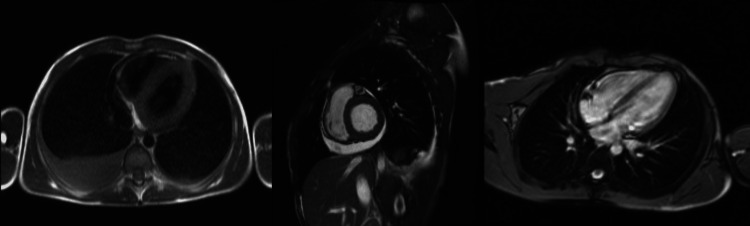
Cardiac magnetic resonance imaging with acute myocarditis
